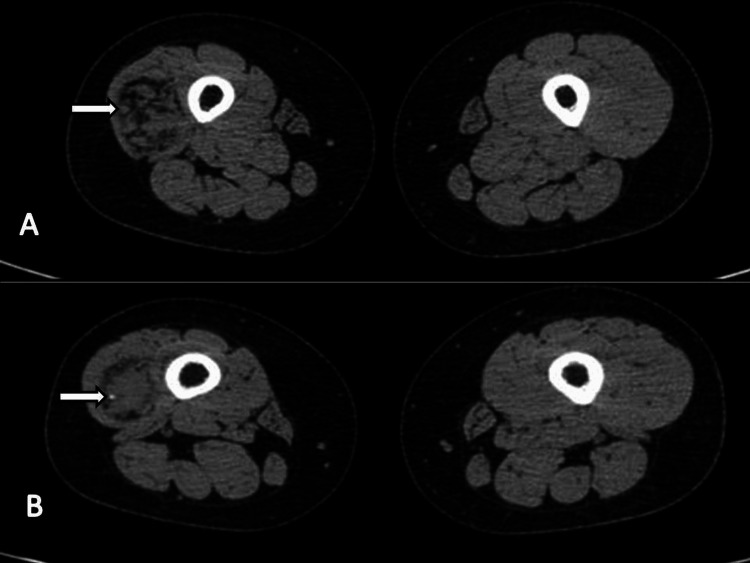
Non-contrast CT images The images show circumscribed heterodense lesion with fat density in intervening spaces on the axial plane (A) and a few calcified phleboliths in the inferior aspect of the lesion (B) CT: computed tomography
